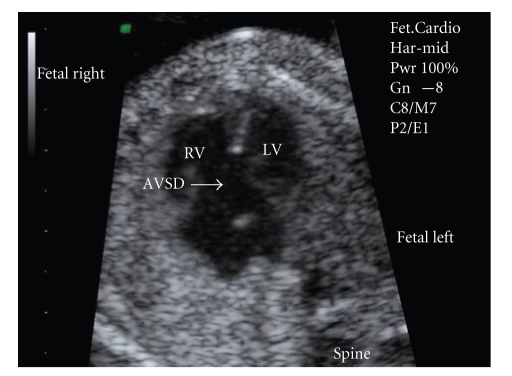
Apical four-chamber view showing a defect of isolated complete atrioventricular septal defet. AVSD: atrioventricular septal defet; RV: right ventricle; LV: left ventricle.
